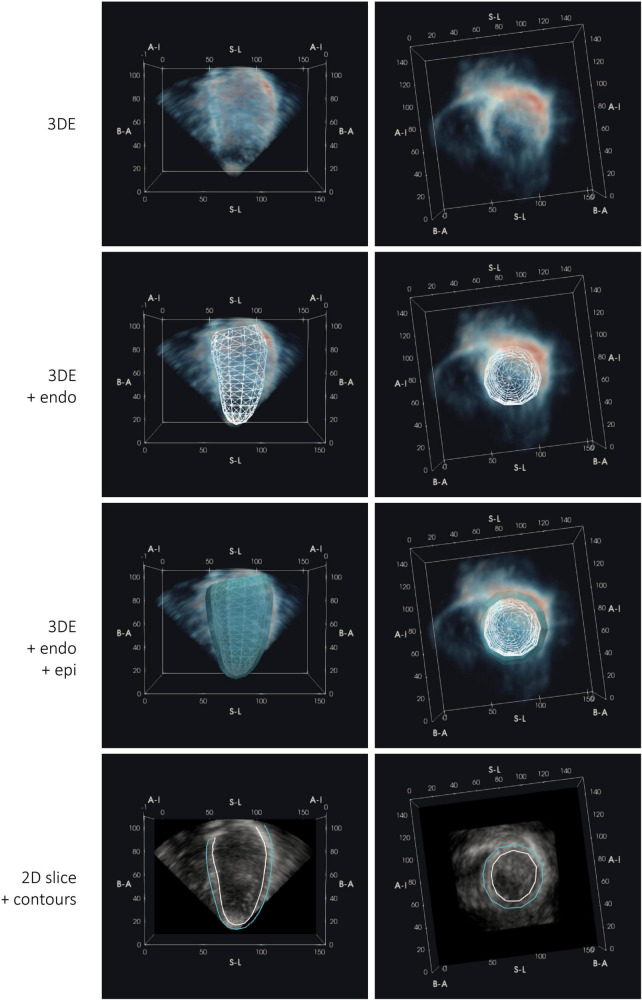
Registration of 3D echocardiography (3DE) with subject-specific geometries of the left ventricle (LV) derived from cardiac magnetic resonance (CMR) at end-diastole, showing an example 3DE image volume (visualized with an opacity transfer function on a blue-to-red colormap) and corresponding 2D mid-ventricular image slice and contours of the endocardium (endo) and epicardium (epi), viewed longitudinally and axially. Labels denote anatomical LV aspects: B-A, base-to-apex; S-L, septal-lateral; A-I, anterior-inferior. All dimensions are in mm.
